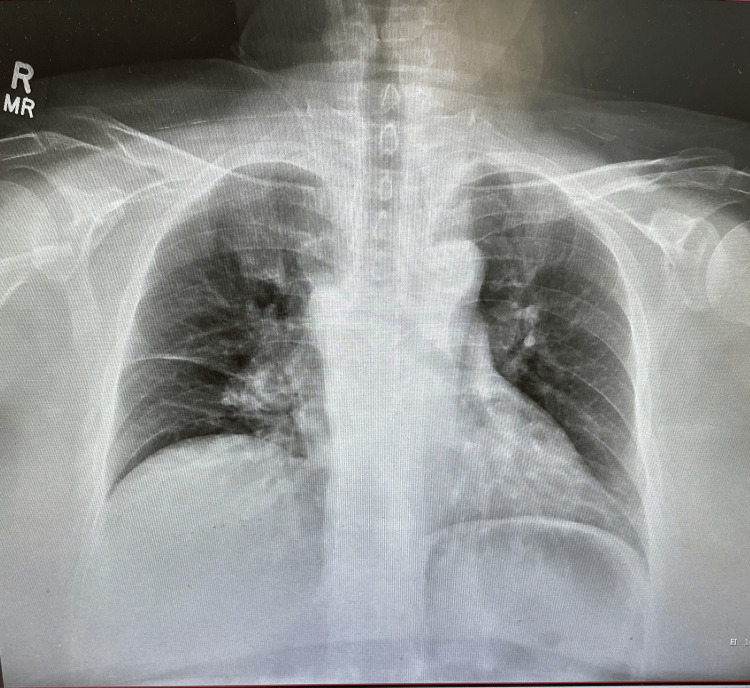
Chest X-ray of the patient showing prominence in the hilar region, an elevated right dome, and atelectasis in the right mid-lung
